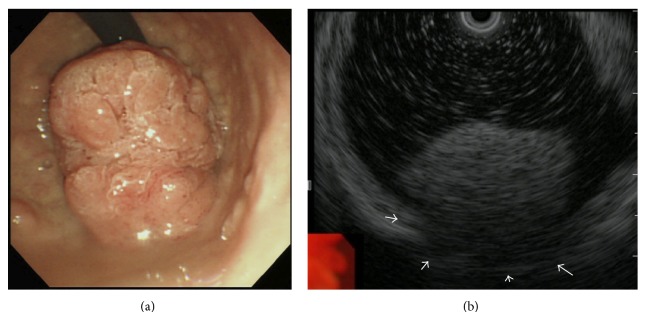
(a) Upper gastric endoscopy revealing a giant polypoid-type tumor extending from the duodenum bulb to the pyloric ring. (b) An endoscopic ultrasound test suggesting that the tumor might invade the muscular tunic (white arrows).
